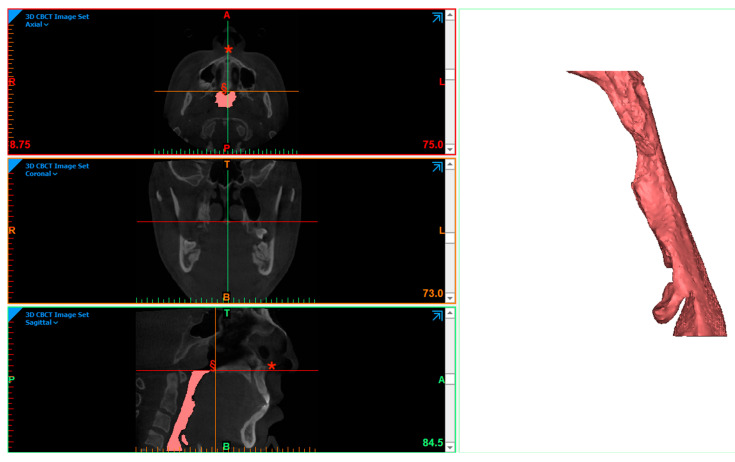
PAS segmentation session on CBCT; *: ANS; §: PNS (patient N°4).
